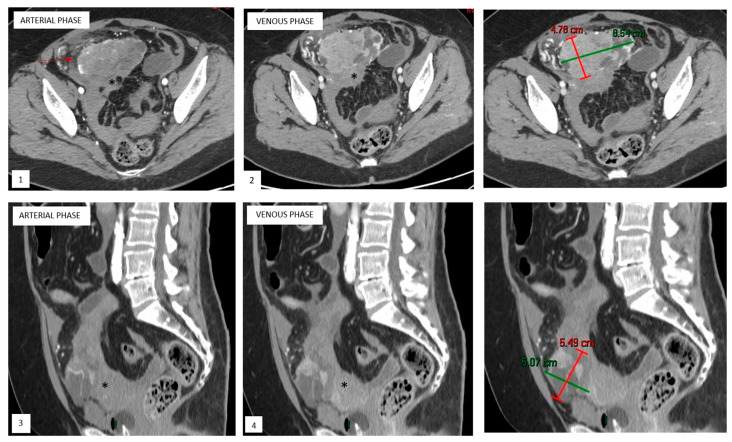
Axial and sagittal reformatted contrast-enhanced CT pelvic image of ruptured uterine PEComa with hemoperitoneum. Axial and sagittal reformatted contrast-enhanced CT pelvic images reveal a well-marginated mass arising exophytically from the uterine fundus (*), with hypoattenuating areas consistent with cystic/necrotic changes and solid components showing progressive heterogeneous enhancement during arterial ((1,3)) and venous ((2,4)) phases. A small amount of peritoneal fluid can also be noted (arrow).
